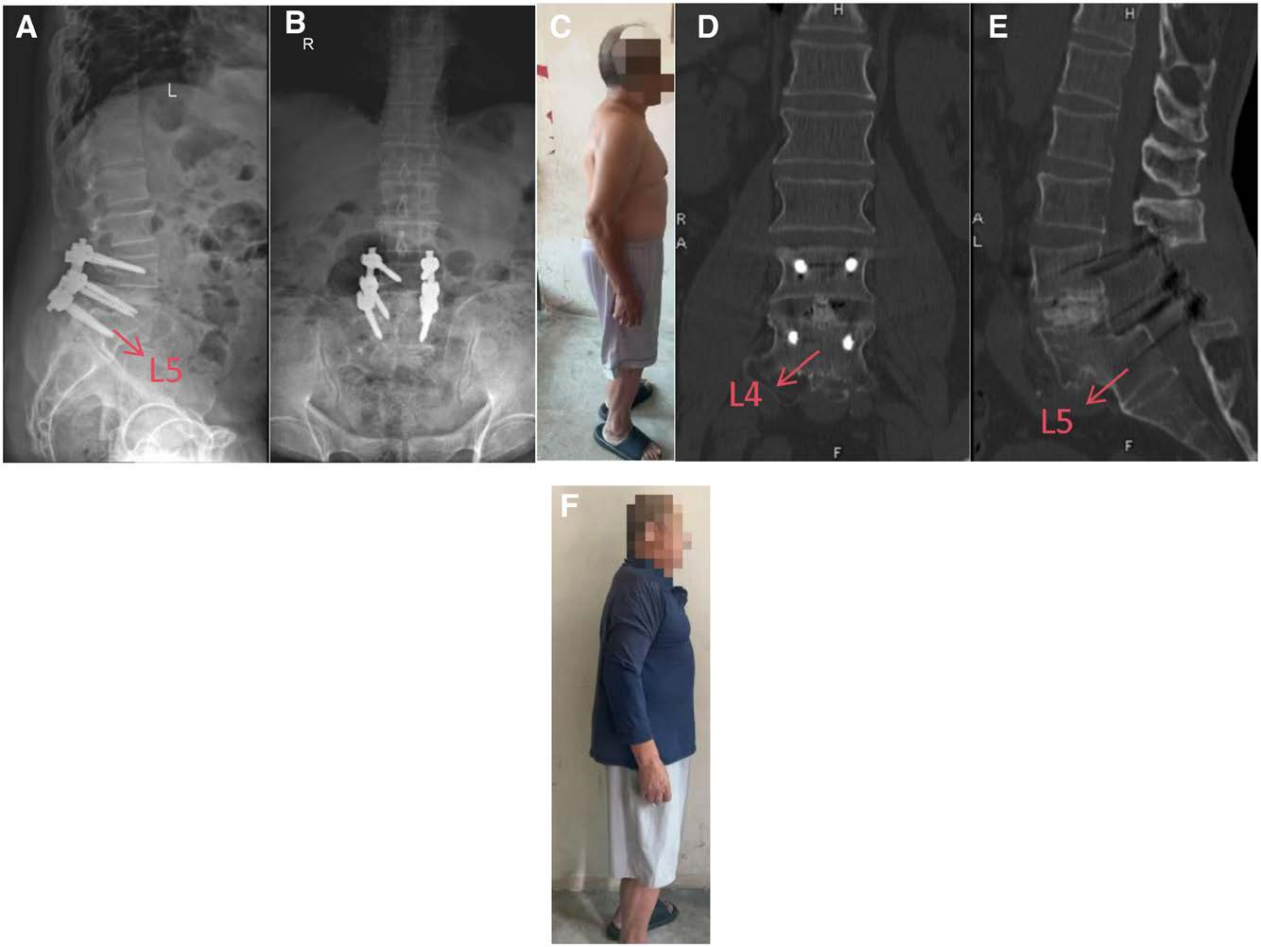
(A–B) Radiographs demonstrate appropriate placement of the screw–rod instrumentation with stable fixation in situ. (C) Shows resolution of the patient’s sagittal anterior trunk inclination, indicating surgical success. (D and E) CT demonstrates early graft incorporation at L3–L4; the pedicle-screw–rod construct is well positioned with fixation maintained in situ. (F) shows the patient’s upper-body forward-lean angle at 0°, indicating a marked short-term postoperative effect.
